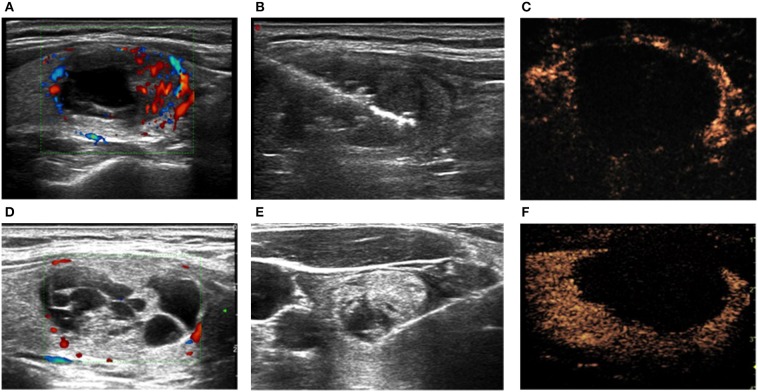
BTNs (A,D) before, (B,E) during, and (C,F) immediately after ablation in the (A–C) LA and (D–F) MWA groups. (C,F) Contrast-enhanced ultrasound.
